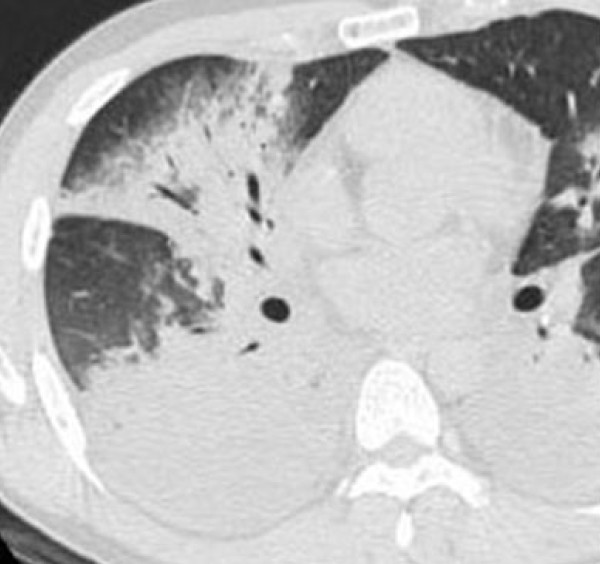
31-year-old man with severe *M. pneumoniae *pneumonia with respiratory failure requiring mechanical ventilation. CT shows bilateral air-space consolidation and pleural effusion.
